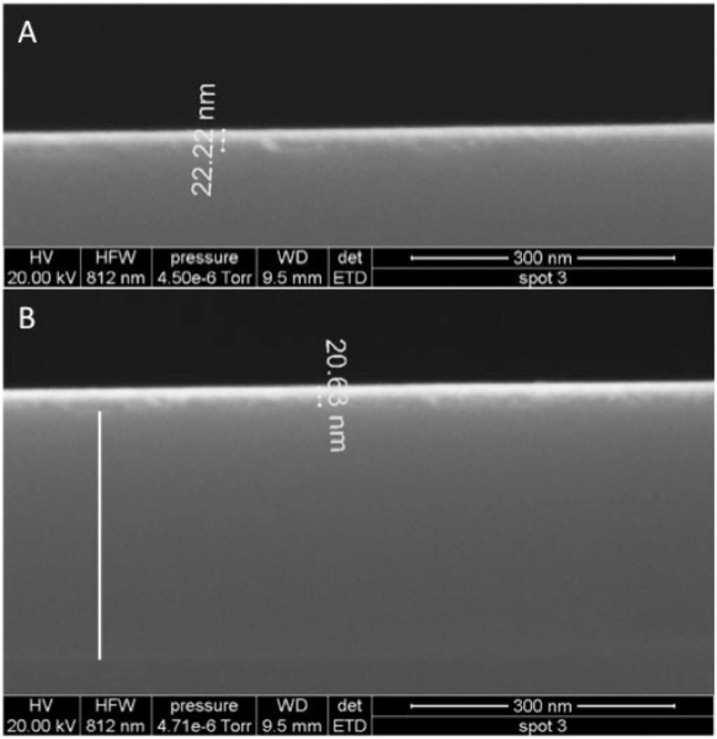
Field emission SEM images of ZTO waveguide coatings. (A) A 22–23 nm ZTO coating (composition confirmed using energy dispersive X-ray analysis, data not shown). (B) A 21 nm coating made on a waveguide; the white line shows the thickness of the waveguide material.
